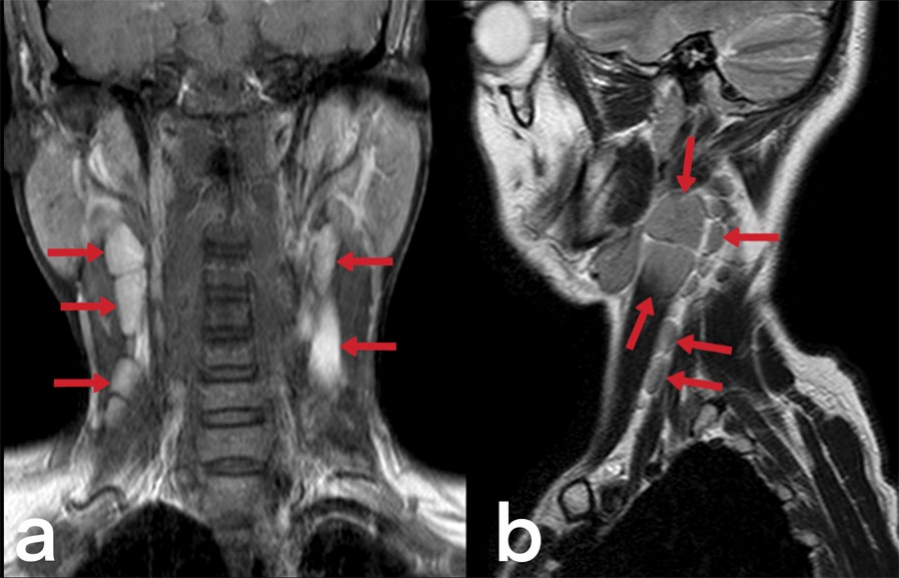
Contrast-enhanced T1 weighted coronal (a) and sagittal T2 weighted (b) MR images of a 39-year-old female demonstrate multiple lymphadenopathies in the bilateral cervical chains. The patient was diagnosed with tuberculous lymphadenitis
